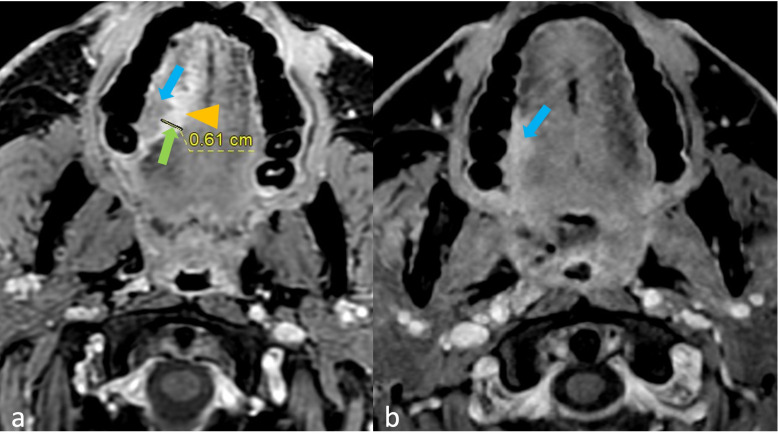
(a, b) Oral tongue squamous cell carcinomas with and without peritumoral hyperenhancement (PH) on MRI. (a) Axial thin contrast-enhanced (CE) fast spoiled gradient echo (FSPGR) sequence shows PH (arrowhead) surrounding the isoenhancing tumor (blue arrow). Measurement of maximum width of PH is 6.1 mm (green arrow), which is the PH width. (b) Axial thin CE FSPGR sequence shows an enhancing tumor without any PH (arrow).
